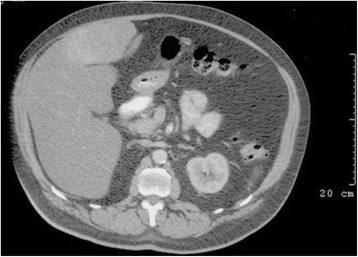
Abdominal CT displaying the pedunculated liver lesion arising from segment IV
